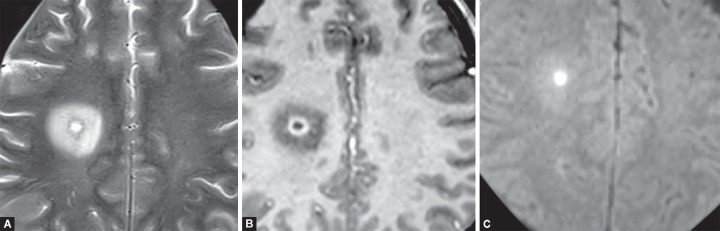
Cerebral abscess. (A) Ring enhancing lesion; (B) in right centrum semiovale of right frontal lobe with T2W hyperintense core, perilesional edema; (C) and central diffusion restriction on DWI image
